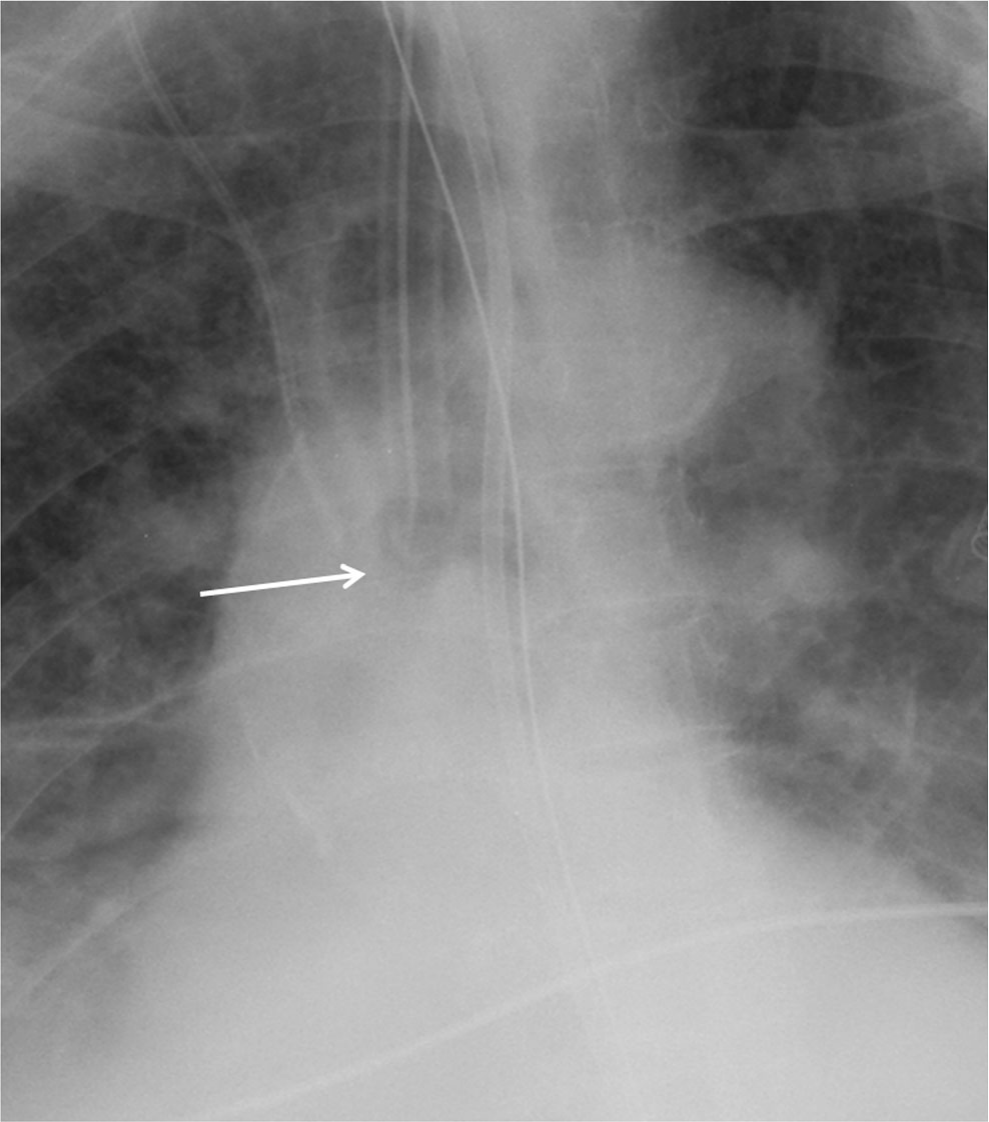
Cropped chest radiograph image centered on the carina. The ET tube tip is low at the orifice of the right main stem bronchus (*white arrow*)
